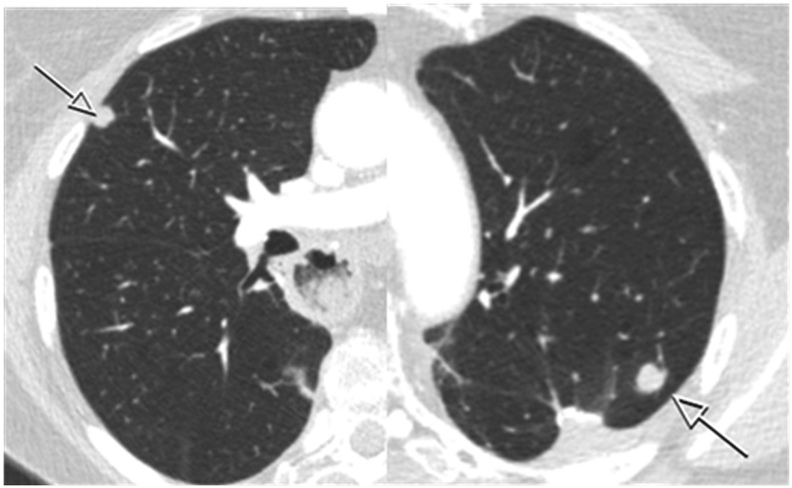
A CT scan 12 months after RAMIE demonstrating a 7.0 mm pulmonary metastasis in the right middle lobe and a 7.0 mm pulmonary metastasis in left upper lobe (indicated by the arrows).
